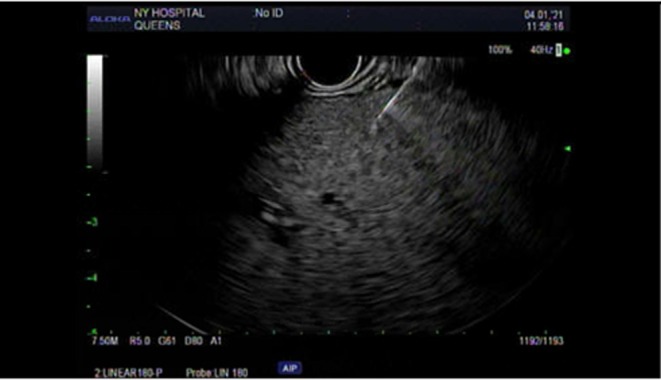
Endoscopic ultrasound view of the liver, displaying a diffuse, abnormal, and heterogeneous echotexture throughout the liver parenchyma, consistent with significant hepatic inflammation.
